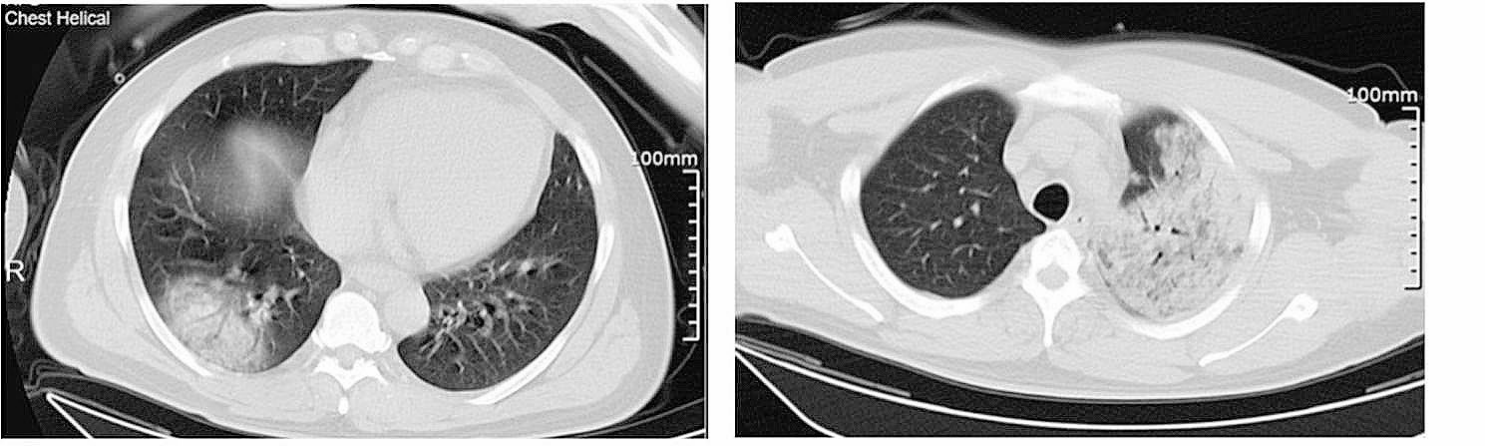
Chest computed tomography scan at admission
